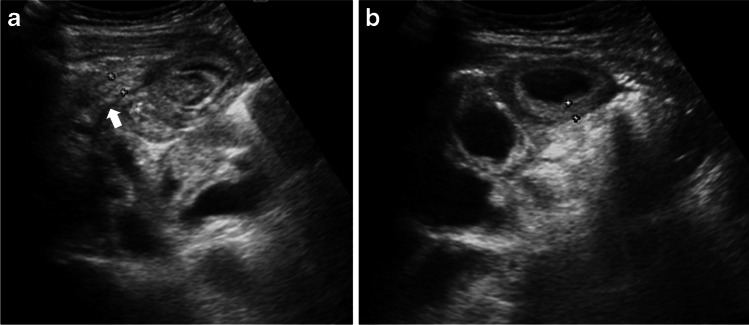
Case 23: a 7-year-old boy with abdominal pain, vomiting and fever. a, b US abdomen transverse section of right iliac fossa shows diffusely thickened multiple small bowel loops (*calipers *in b) with free fluid and mesenteric inflammation. The appendix is mildly inflamed (7 mm *arrow*). CT scan on day 4 of admission (not shown) showed similar findings as US, in addition to enlarged kidneys which had increased in size compared to initial US
